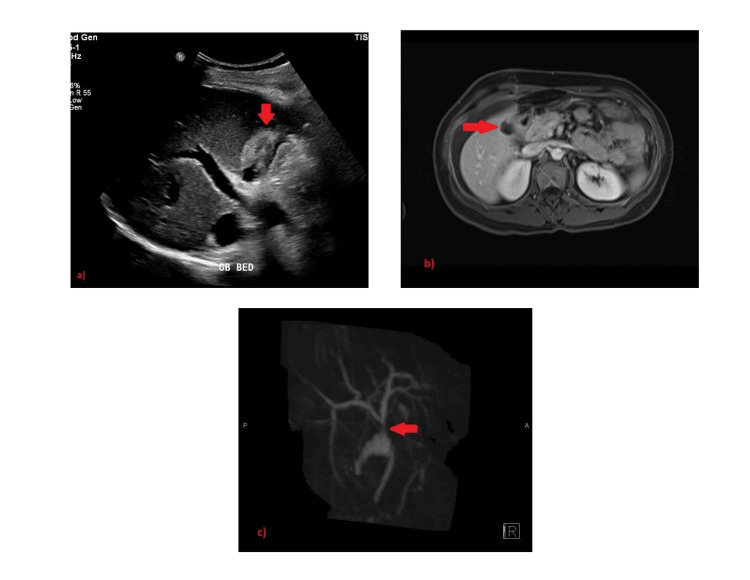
Selected images of ultrasound and magnetic resonance cholangiopancreatography showing gallbladder fossa collection. (a) Ultrasound abdomen shows a well-defined collection within the gallbladder fossa (arrow). (b) Axial T1 fat sat post-contrast axial image shows gallbladder fossa collection, with rim enhancement (arrow). (c) Coronal 3D reconstruction of magnetic resonance cholangiopancreatography image showing suspected communication between the collection’s upper part and adjacent hepatic duct confluence (arrow), concerning bile duct injury.
